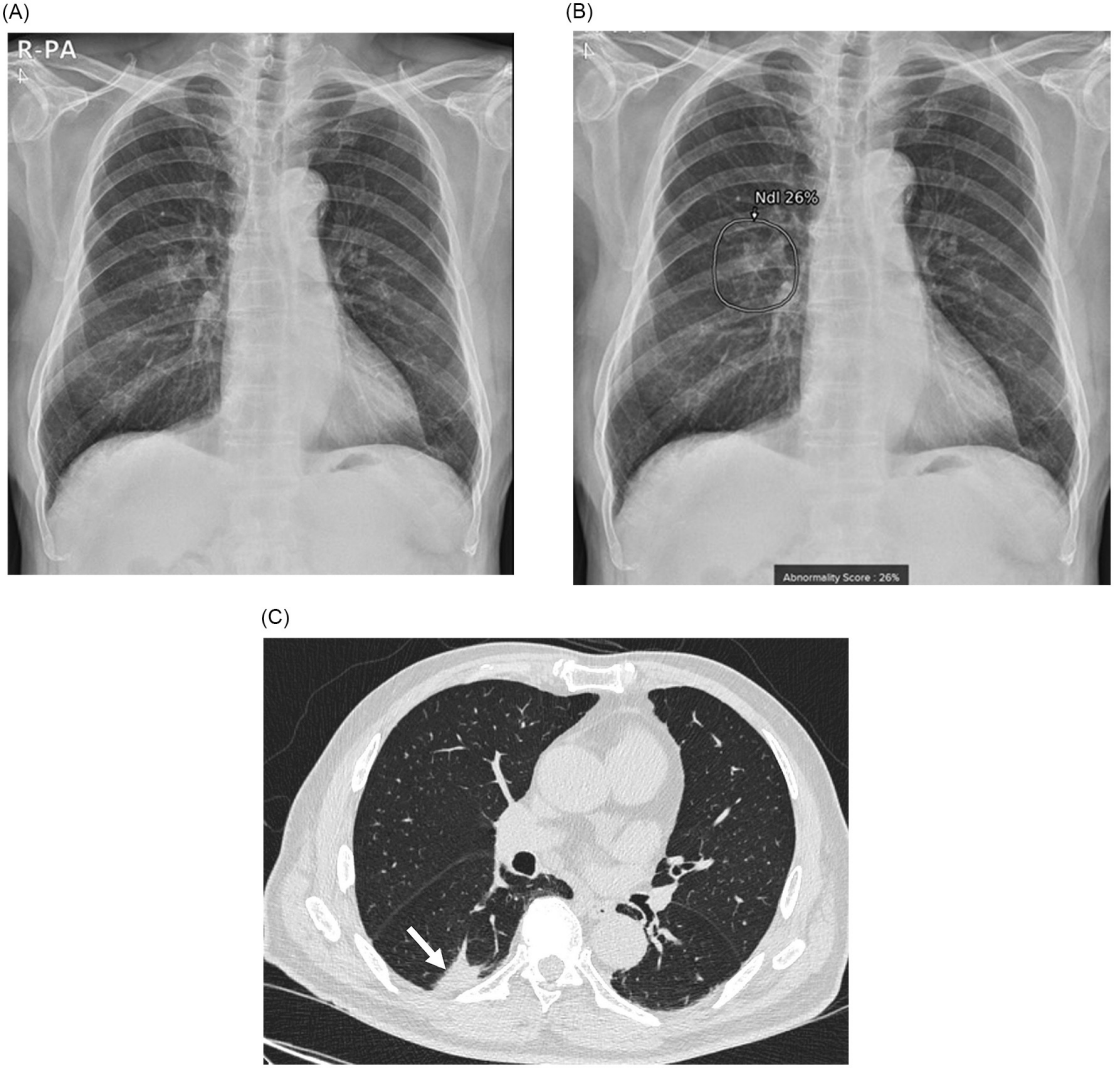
Chest radiograph and computed tomography (CT) of a 79-year-old man (#13 patient). (A) Chest radiograph was taken in the cardiomegaly outpatient clinic for the evaluation of arrhythmia. (B) Artificial intelligence-based lesion detection software detected a lung nodule in the right lower lobe with an abnormality score of 26%. (C) Chest CT demonstrated an irregular solid mass in the posterior subpleural space of the right lower lobe superior segment (arrow). After surgical resection, adenocarcinoma with an approximate size of 2.8 cm and the final stage of IA3 (pT1cN0M0) was confirmed.
